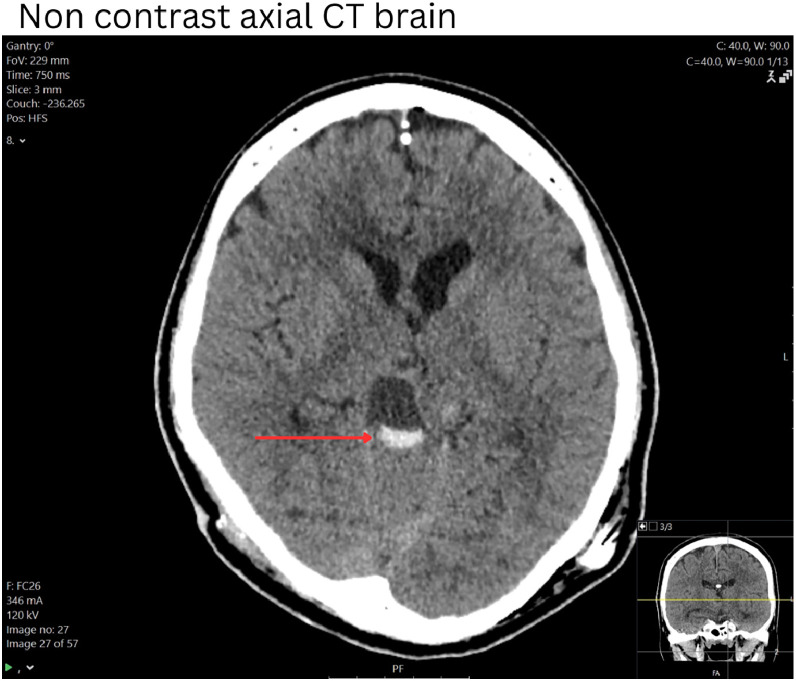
Non contrast axial CT image of the brain demonstrating hemorrhage into the midbrain tectal plate lesions following insertion of a ventriculoperitoneal shunt (not shown). The ventricles are decompressed.
